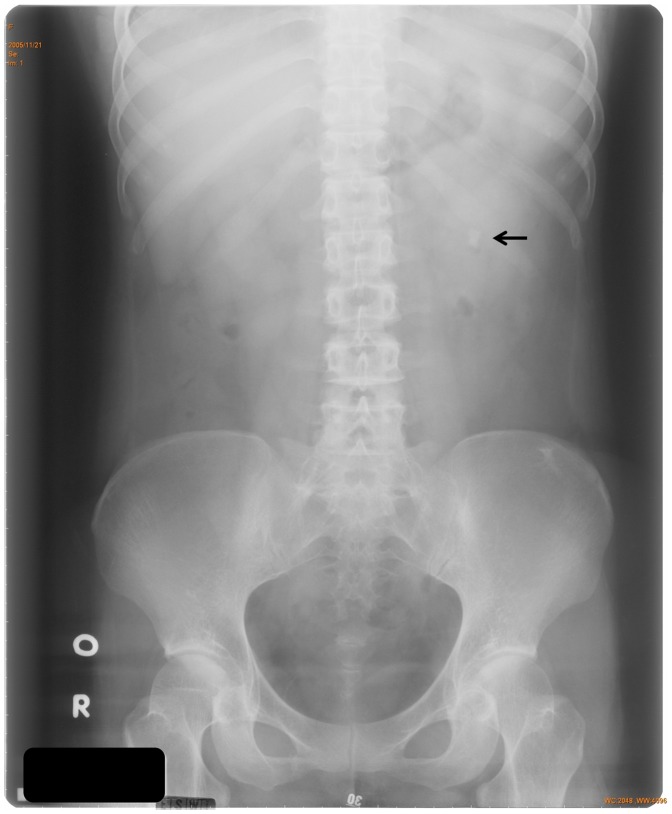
Radiography of the kidneys and urinary bladder. A radio-opaque stone measuring 11×6 mm in size in the central portion of the left kidney (arrow).
